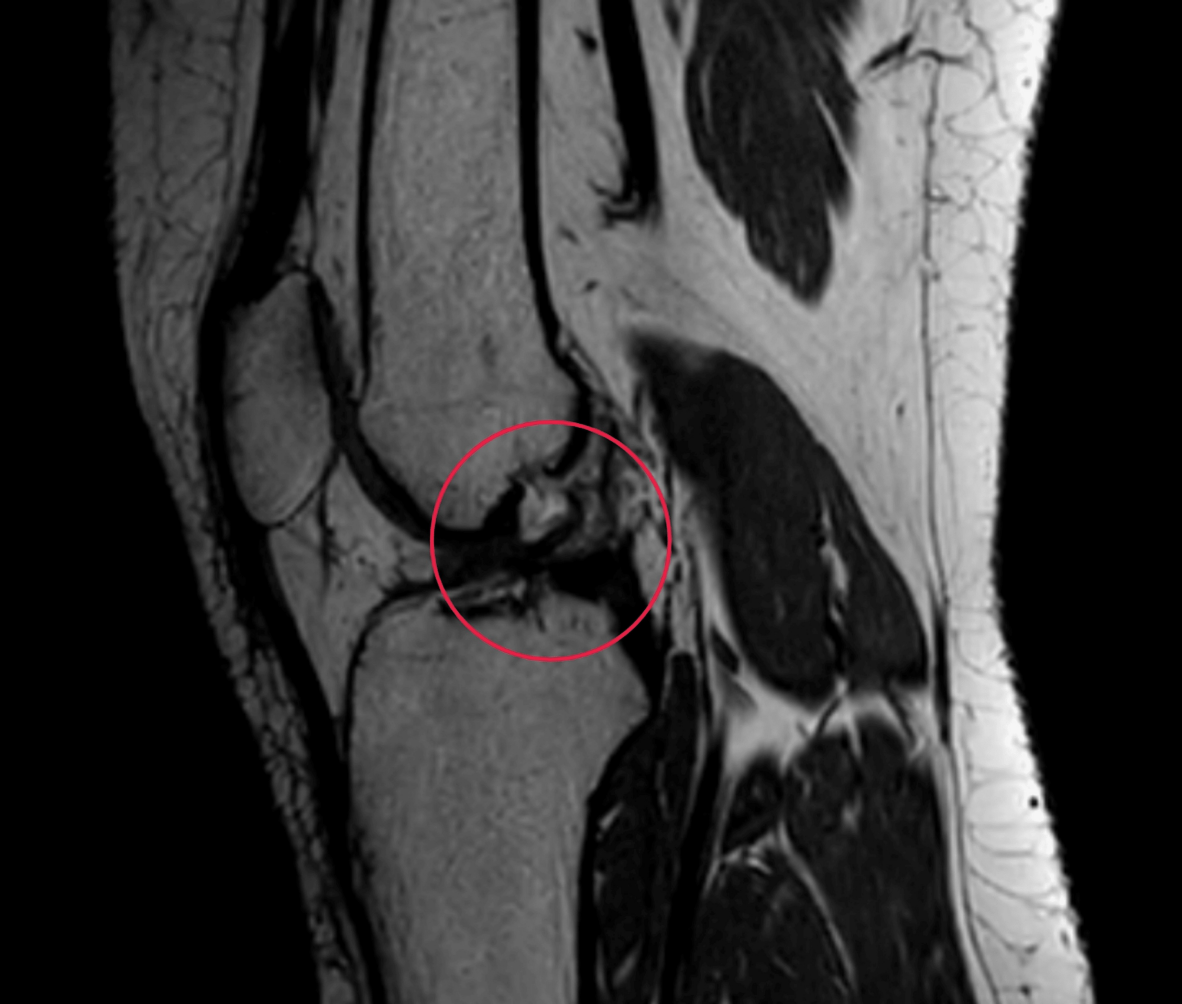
An MRI of the right knee showing a complete-thickness ACL tear The circle represents a complete-thickness ACL tear MRI, magnetic resonance imaging; ACL, anterior cruciate ligament
